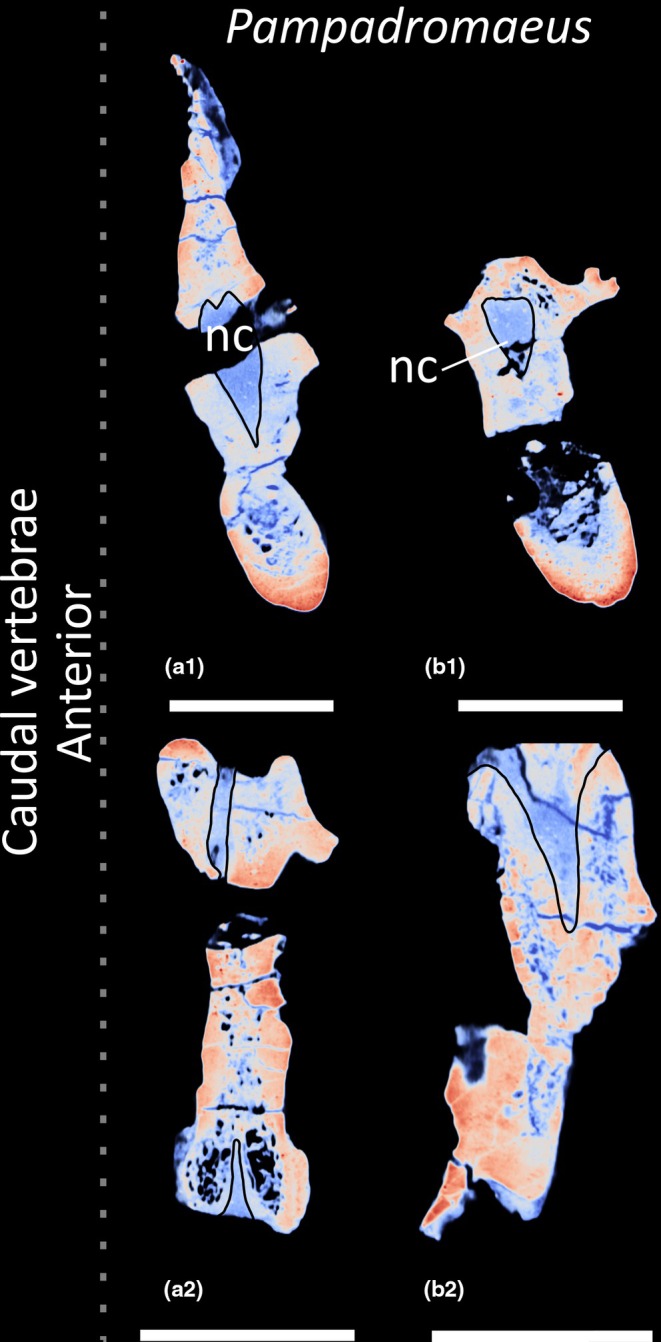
Colour density map outlining PSP in CT scan slices of the anterior caudal vertebrae of *Pampadromaeus barberenai*. (a1, b1) Transverse cross‐section, (a2, b2) horizontal cross‐section. nc, neural canal. Scale bars = 2 cm.
